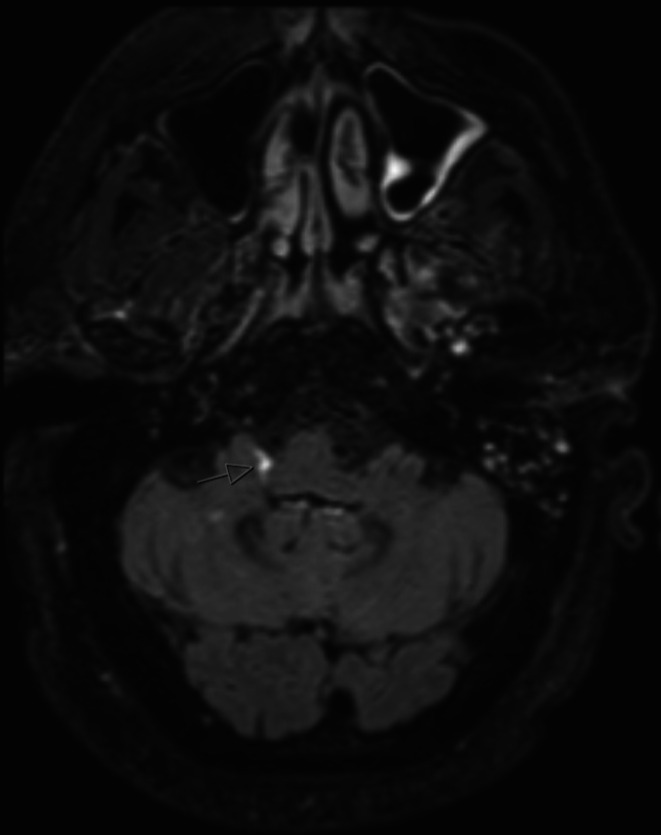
MRI brainstem post gadolinium T2 FLAIR demonstrating leptomeningeal enhancement (arrow) on the right side of the medulla extending into the root of the vagus nerve.
